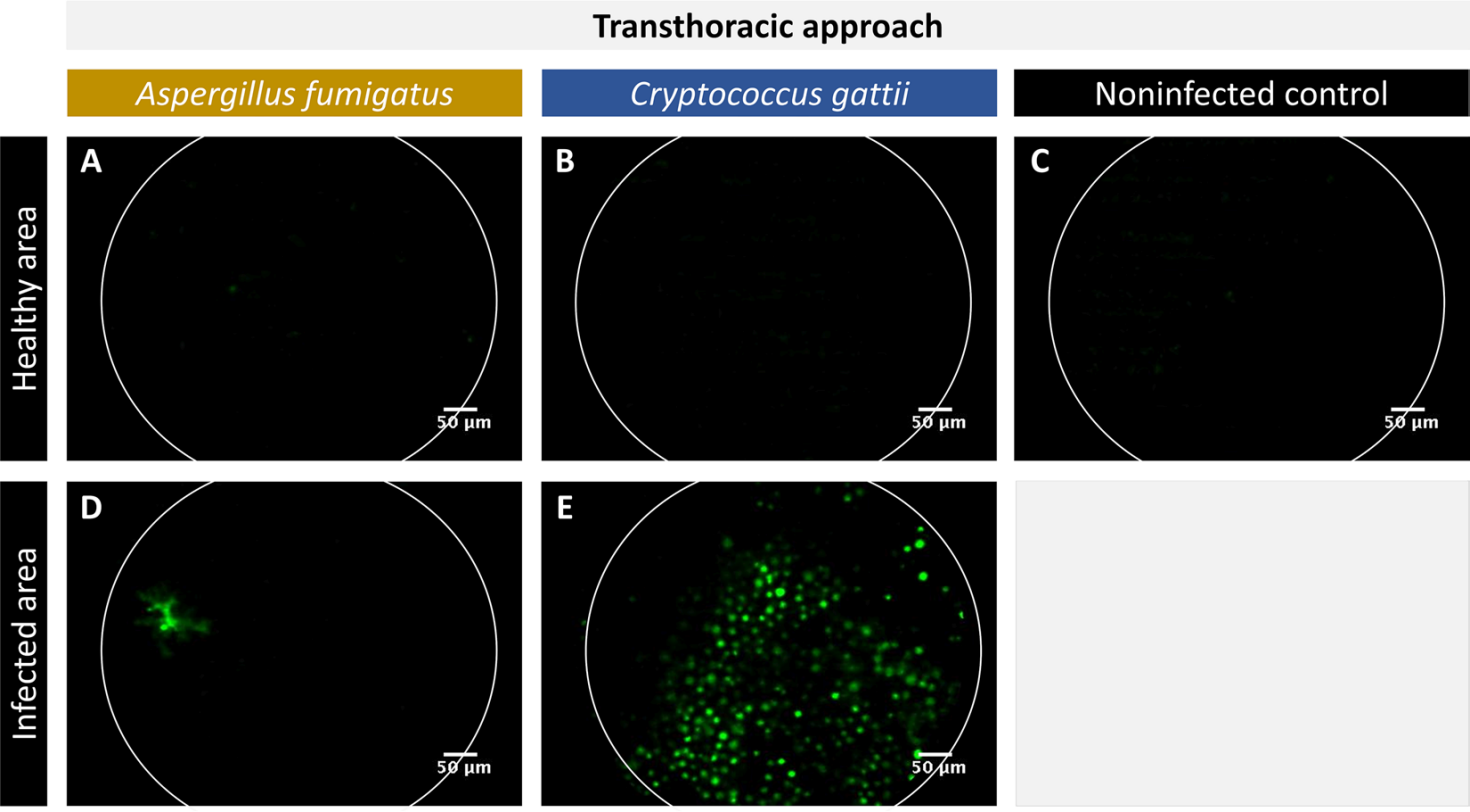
In situ*, transthoracic FCFM of lung infections. The images were acquired by placing the fibre-optic probe (S-1500) into direct contact with the lung surface after performing a bilateral thoracotomy. (A–C) Representative FCFM images of a healthy appearing area of an *A*. *fumigatus* (GFP-expressing) infected lung, a *C*. *gattii* (GFP-expressing) infected lung and a non-infected control lung. (D,E) Representative FCFM images of an infected area of an *A*. *fumigatus* infected lung and a *C*. *gattii* infected lung, showing the presence of fungal cells.
